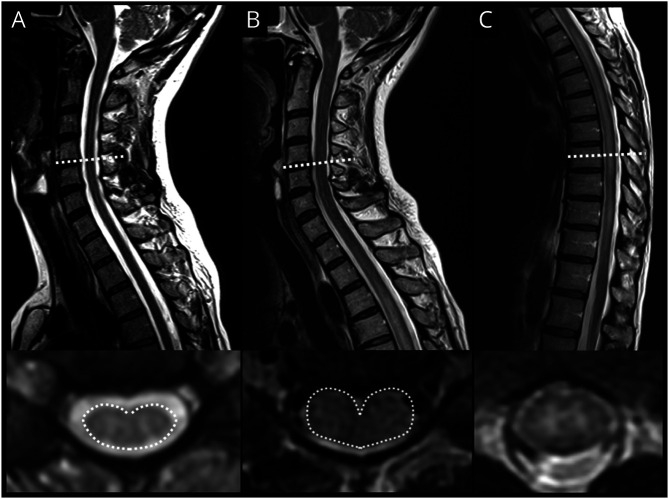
Spinal MRI in Subacute Rapidly Progressive HAM With Severe Spinal Cord Swelling Sagittal (top) and axial (bottom) T2w images 10 years before the presentation (A) and at presentation (B and C) in a 55-year-old woman with a 2-month history of rapidly progressive spastic paraplegia and bladder dysfunction. Imaging at nadir (B and C) demonstrates a new long-segment high T2w signal in the central thoracic spinal cord and diffuse swelling, which involves the entire spinal cord extending beyond the region of abnormal signal with a “heart symbol”–like appearance to the cervical spinal cord (B, bottom image).
